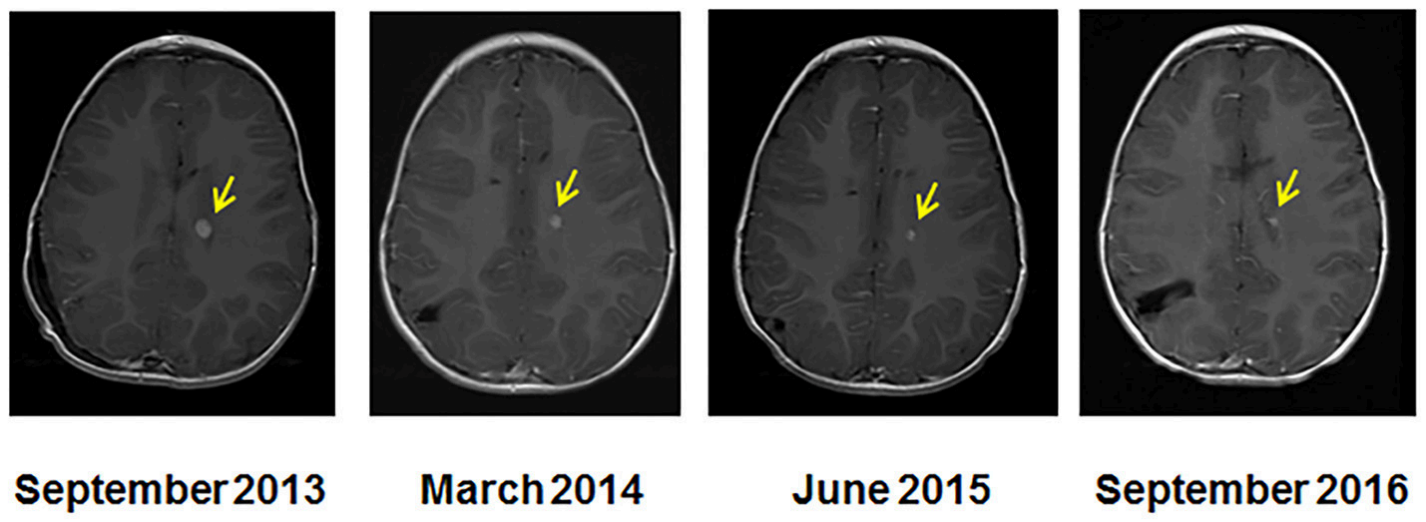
Serial MRI imaging of the CPC patient. MRI at the start of molecular guided therapy regimen (left, Sept 2013), at 7 months (March 2014), at 20 months (June 2015), and at 36 months (September 2016). The arrows indicate the location of the tumor and size reduction over the course of treatment.
